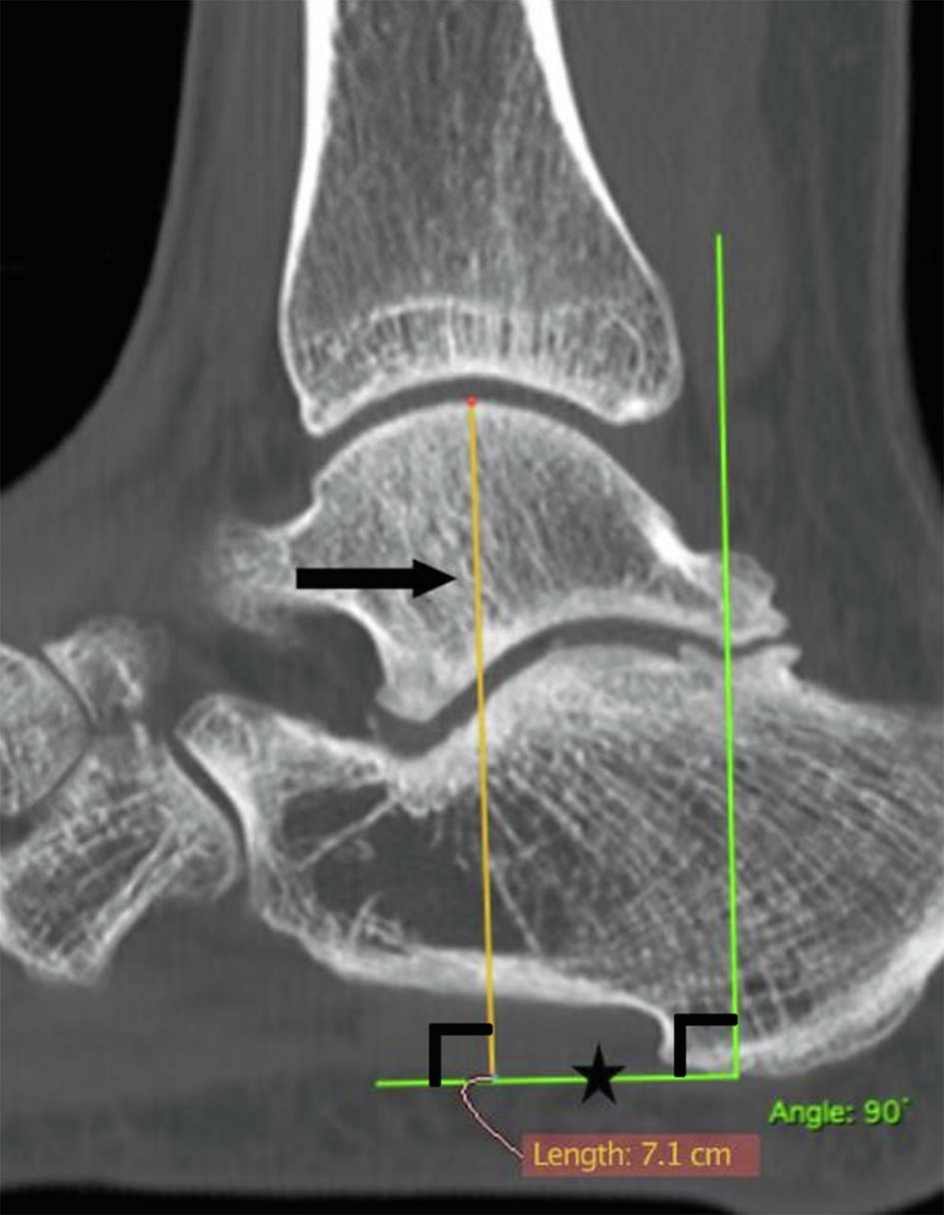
Preoperative hindfoot height (HFH) CT scan measurement. *Plantar fascia,* ⋆*; HFH, → .*
